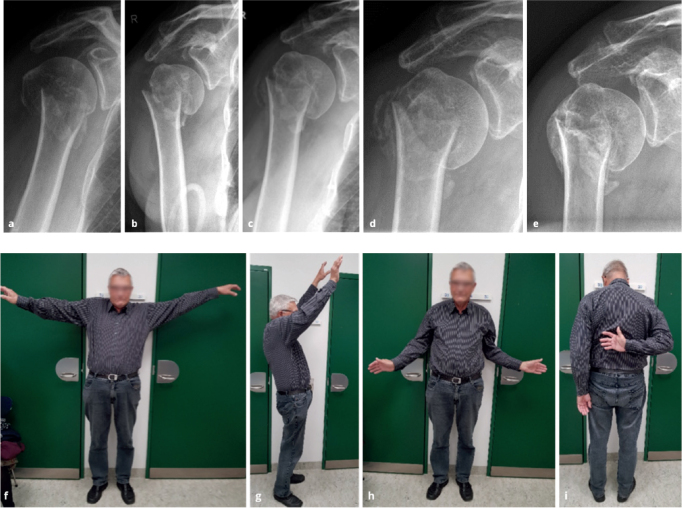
Right 2-part surgical neck fracture in a 73-year-old male. Progressive varus collapse of the humeral head occurred during the first 6 weeks. Solid healing and pain-free function at shoulder level were obtained. Anterior–posterior radiographs at admission, 1, 3, 6, 12, and 24 weeks (a–e). Photos 24 weeks after injury (f–i). At 24 weeks, OSS was 42 (86%), and EQ-5D-3L was 0.78
